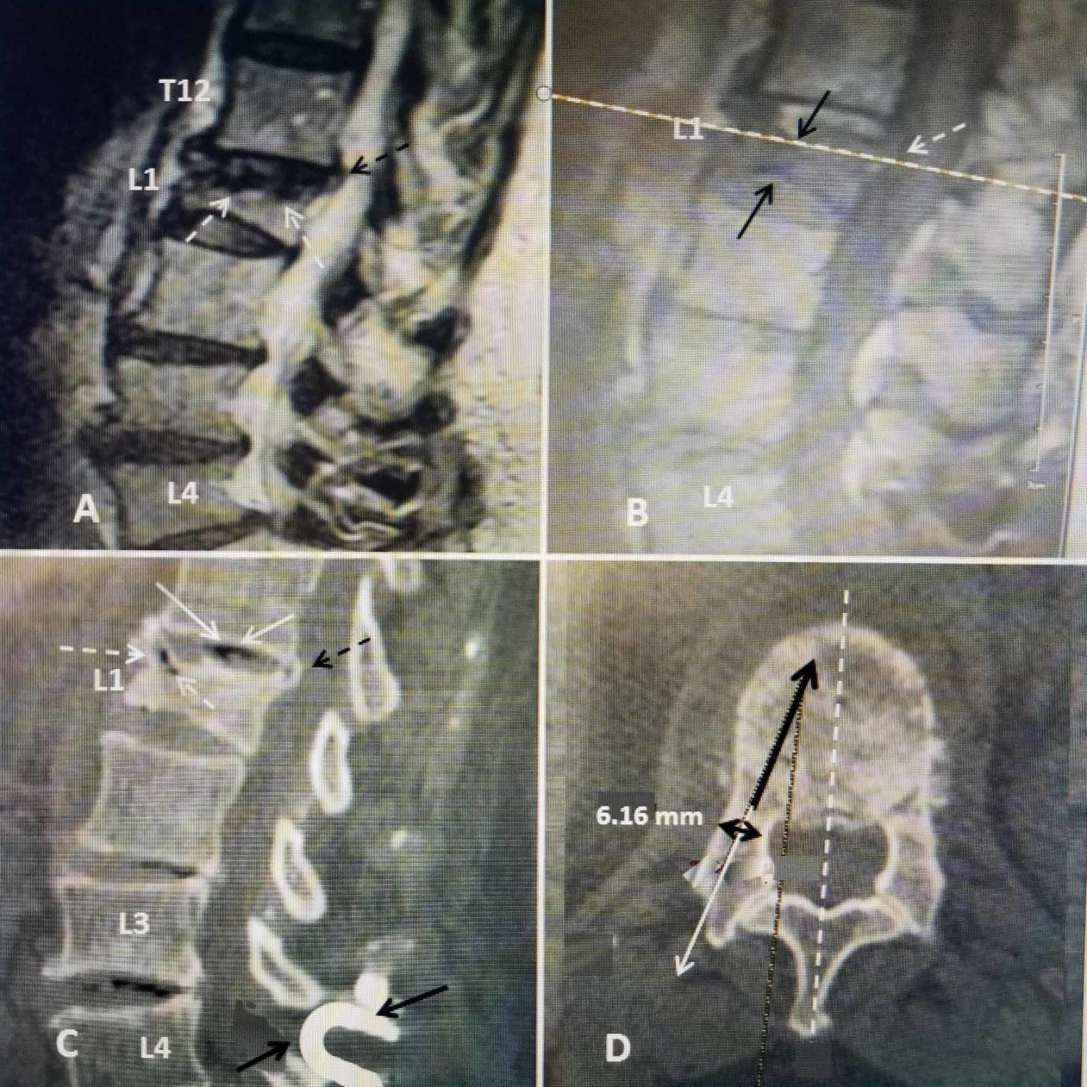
Pre-operative films of acute osteoporotic compression fracture at L1 A: Initial sagittal T2 MRI showing over 50% collapse of the L1 middle and anterior vertebral body (dashed white arrows) with minimal 2-mm displacement of the posterior part of the superior endplate (dashed black arrow) into the spinal canal. B: Sagittal T1 image showing there is also indentation of the inferior endplate of L1 causing biconcave compression (solid black arrows) and edema in the vertebral marrow. The cut used to measure for the SpineJack^R^ implant is indicated (dashed white line across scan). The 2-mm posterior superior endplate displacement is seen (dashed white arrows). C: Sagittal CT vacuum change within the T12-L1 disc space (solid white arrows) and also within the anterior superior part of the vertebral body under the superior endplate (dashed white arrow). Minimal 2-mm posterior displacement of the superior endplate is seen into the spinal canal (dashed black arrow). The previously inserted Coflex interlaminar device inserted at L4-5 is seen below the fracture (solid black arrows). D: Axial CT view showing the measurement of angle and depth for the implant (solid black arrow). By visualizing the line posteriorly (solid white line with arrow) the trajectory for insertion of the SpineJack^R^ is determined. The internal width (bicortical dimension) of the pedicle is indicated as 6.16 mm (double-headed black arrow) to select the best-closed implant size. The implant is designed to be positioned in front of the pedicle, posteriorly and just off-center of the midline anteriorly bilaterally (dashed white arrow).
